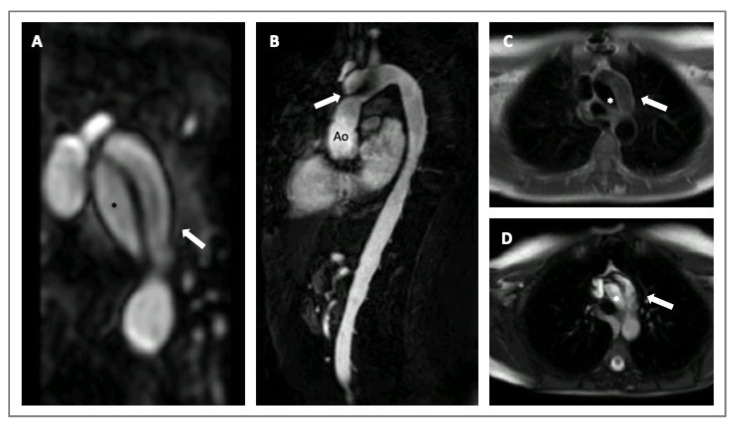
CMR imaging of a 30-years old woman with MS presenting with an acute aortic dissection diagnosed during a routine exam. Angio-MRI with post contrast-enhanced sequence (Panel A); cine-SSFP sagittal and axial images (Panels B,D); T1-weighted axial images (Panel C). The asterisks indicate the true lumen and the arrows the false lumen. Legends: aorta (Ao); steady-state free precession (SSFP).
